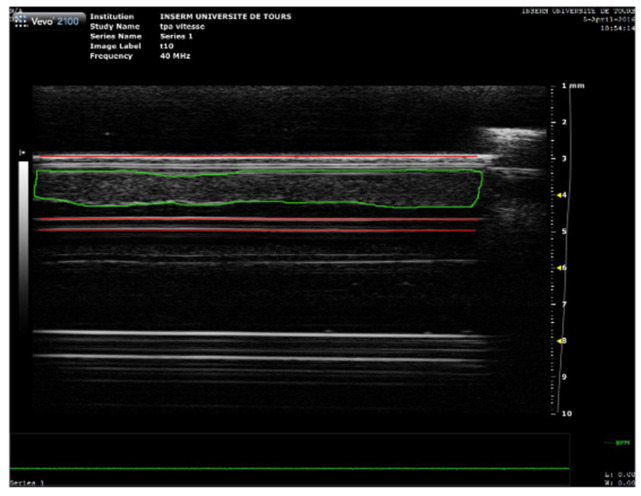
High frequency ultrasound imaging of blood clot. A 2D image of the blood clot obtained with the Vevo 2100^®^. The red lines delineate the anterior and the posterior walls of the tube, while the green shape delineates the clot.
